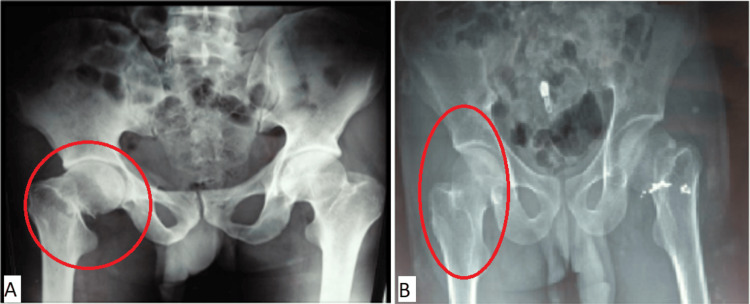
Radiographic examination of two of the patients with intracapsular femoral neck fractures (A) A 25-year-old male having a fracture due to RTA. (B) A 45-year-old female having a fracture due to a self-fall RTA: road traffic accident
